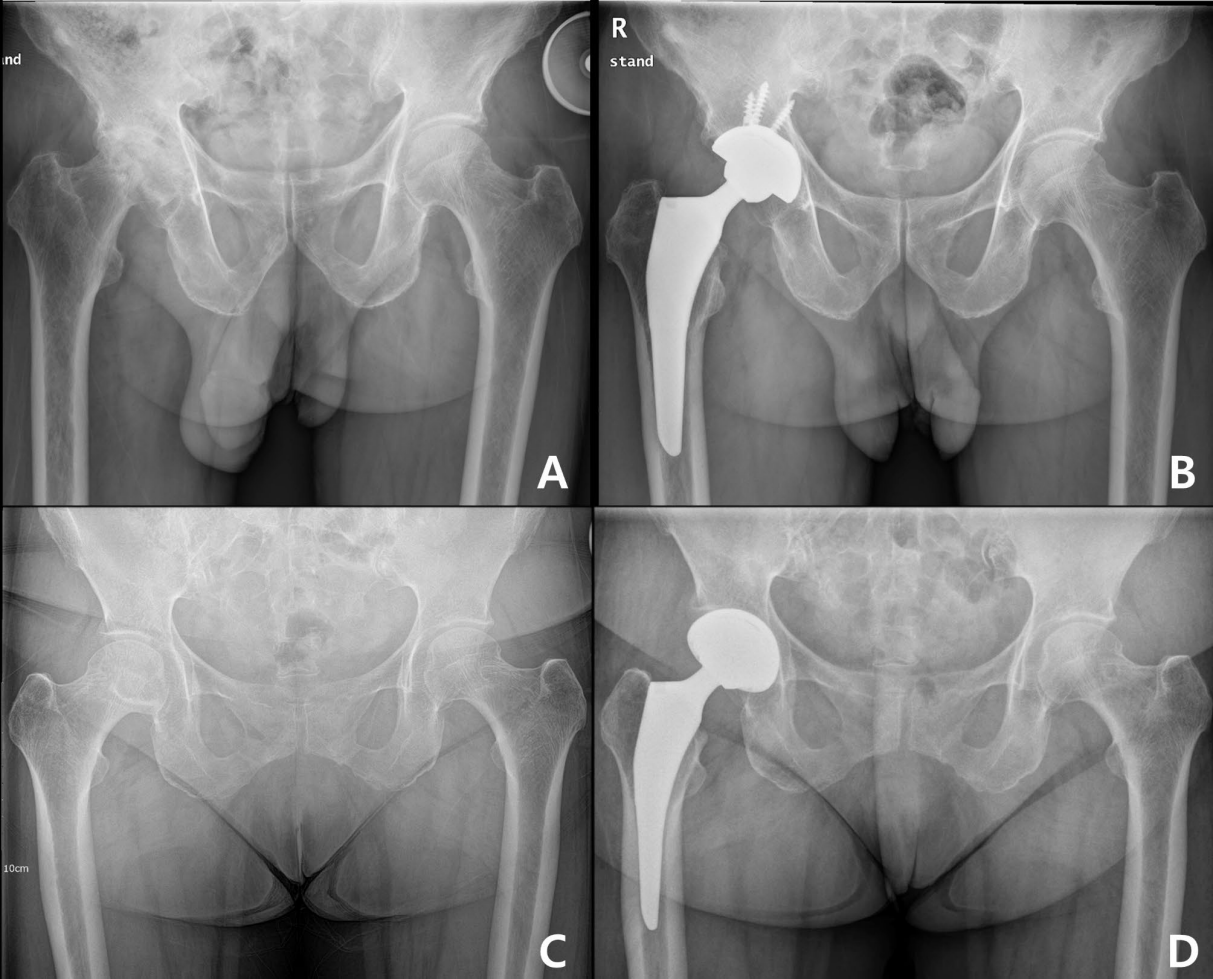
(A) Preoperative hip radiograph with substantial leg length discrepancy (B) Hip radiograph at postoperative 6 weeks with minimal leg length discrepancy (C) Simple hip radiograph with minimal preoperative leg length discrepancy (D) Postoperative 6 weeks radiograph with leg length discrepancy more than 5 mm
